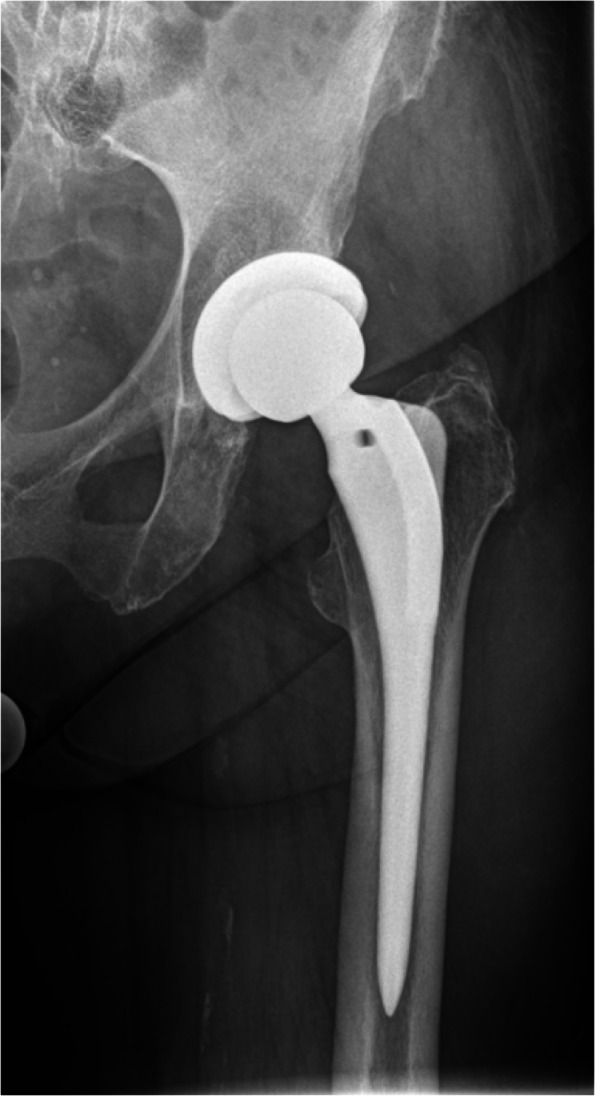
X-ray from a patient suffering from PJI. Plain radiograph showing normal findings
